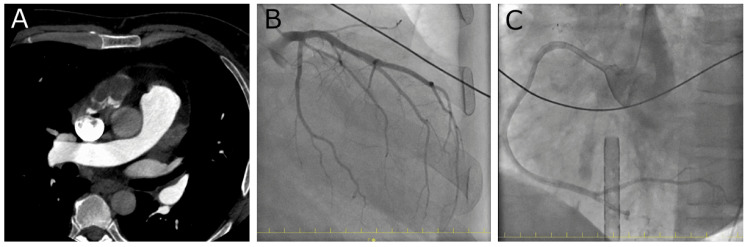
Enhanced computed tomography and coronary angiography findings. (A) Computed tomography findings showed no evidence of pulmonary thromboembolism, although a right atrial thrombus was present. (B, C) Coronary angiography revealed no signs of coronary artery disease.
